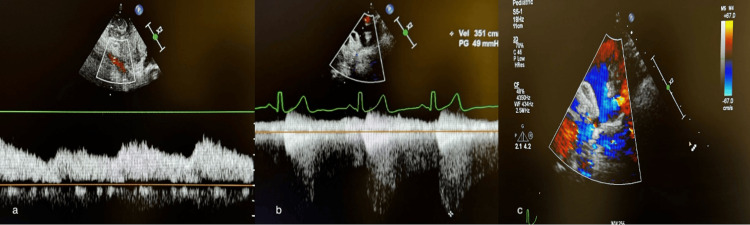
Doppler and color flow echocardiography images (a and b) Spectral Doppler readings — used to measure blood flow velocities across cardiac valves — confirm coarctation of the aorta. (c) Color Doppler echocardiography shows turbulent flow across a valve or septum. The mixed red and blue flow suggests regurgitation.
